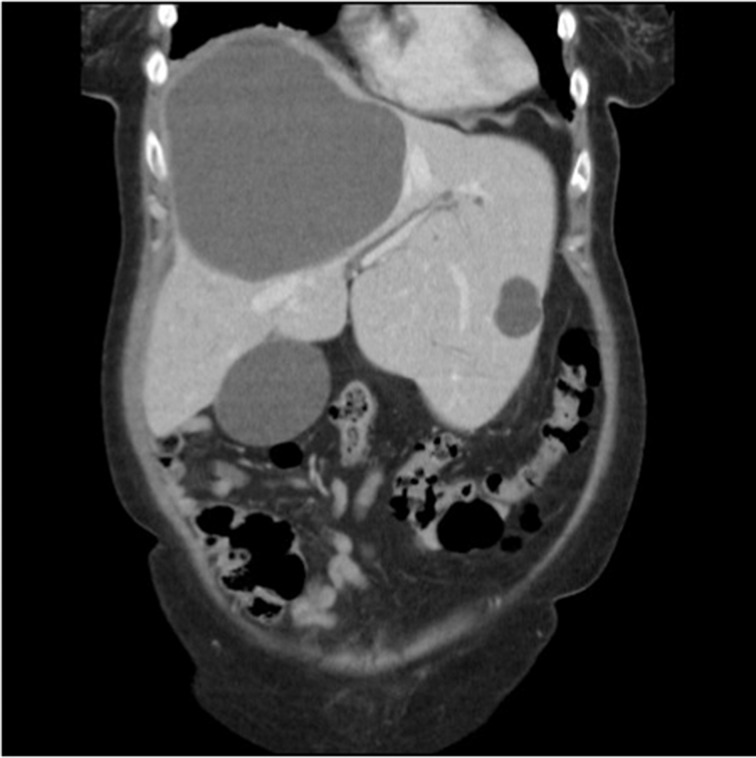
Contrast enhanced computed tomography transverse (left) and coronal (right) section showing 16cm × 16cm cystic lesion in right lobe of liver
